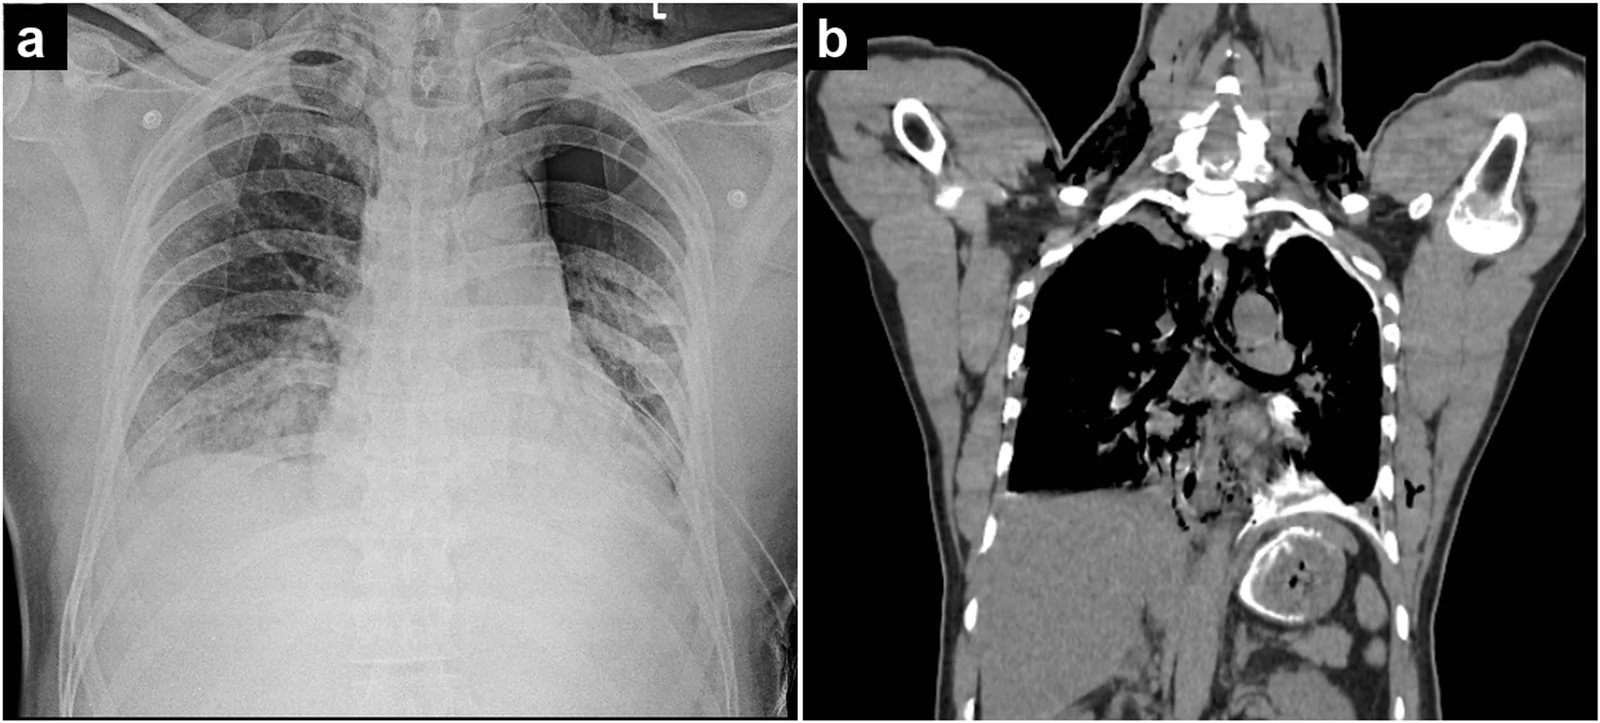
Case 1 Images (a) Chest radiograph showing a left hydropneumothorax with an intercostal drain. (b) Coronal oral-contrast computed tomography showing leak of contrast from the lower oesophagus into the left pleural cavity.
